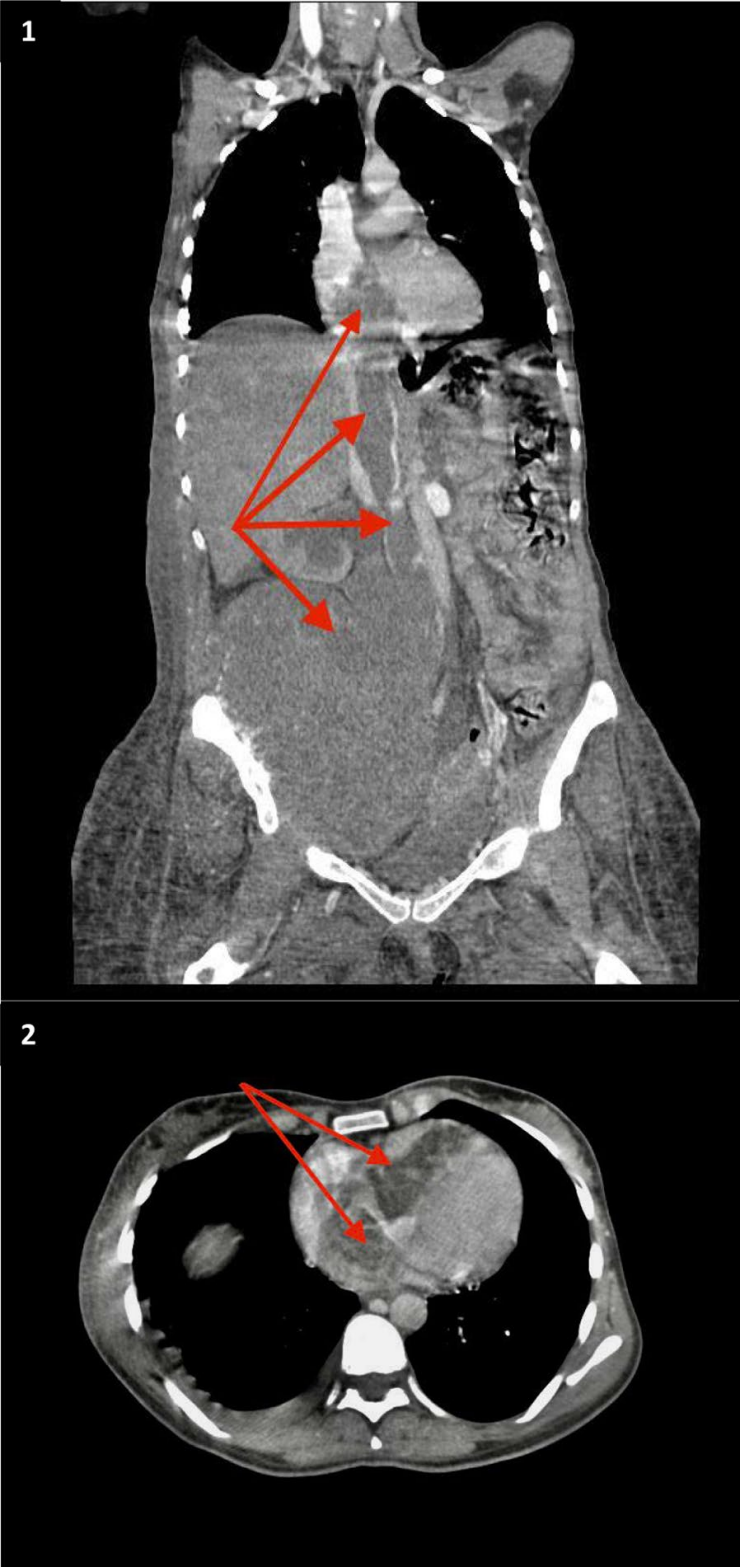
1 Thoraco abdominal angio graphy computed tomography: coronal view: large lesional process of the right coxal bone with endo and exopelvic development associated with bone lysis and invading the IVC which is partially thrombosed with the mass of the same characteristics in favor of a tumoral extention to the right heart chambers. 2 Thoracic angiography computed tomography, transversal view: hypodense mass obliterating right heart chambers. Red arrows: Tumor extension into the IVC and right cavities
